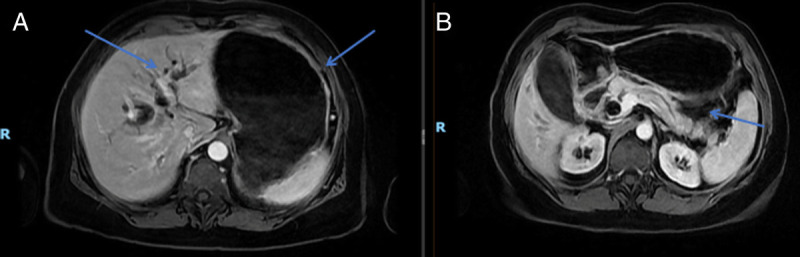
Preprocedural MRI. A, Gastric distention and dilation of intrahepatic bile ducts. B, Dilation of main pancreatic duct. MRI: Magnetic resonance imaging.
